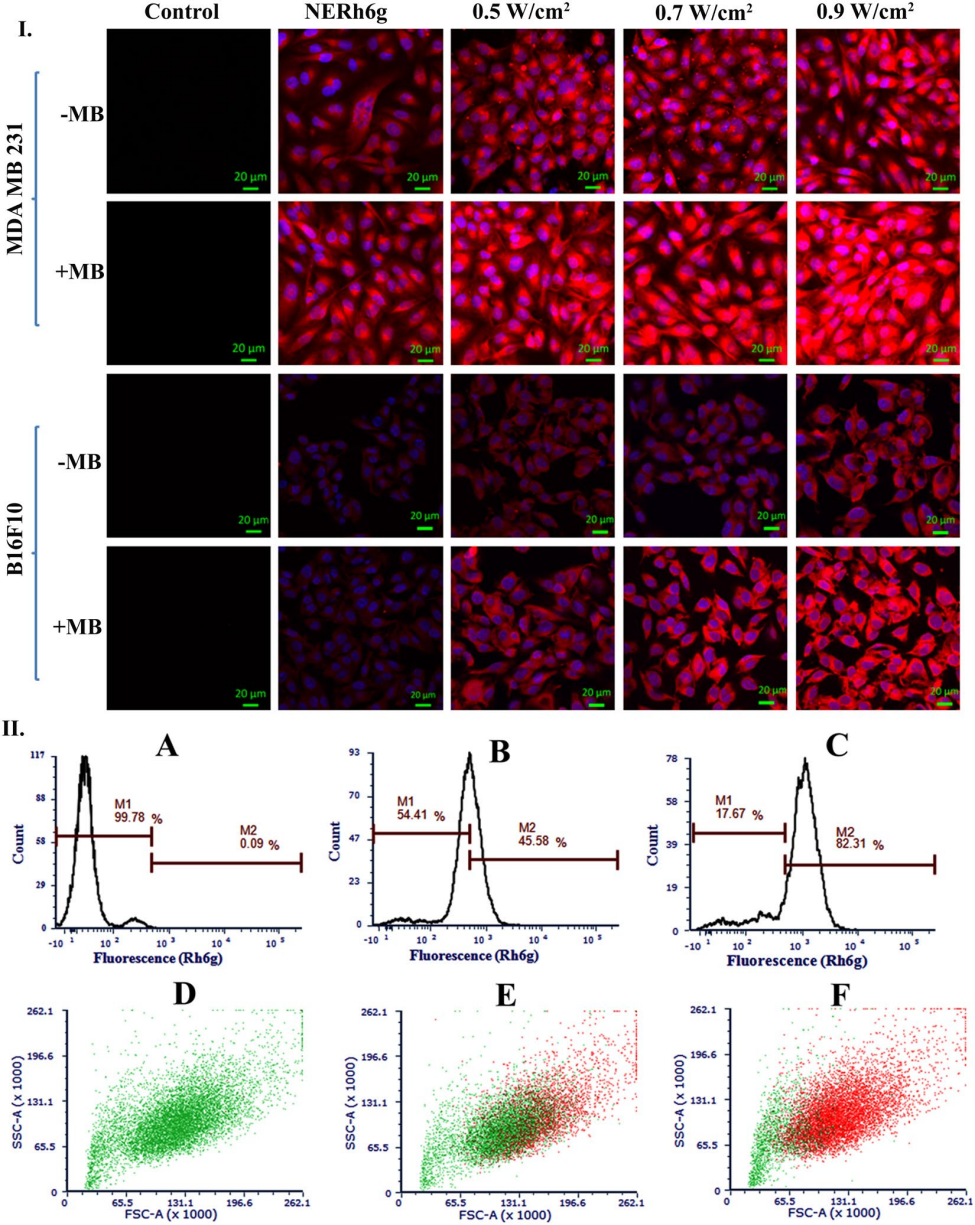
I. CLSM image of NERh6g internalized B16F10 melanoma and MDA MB 231 breast cancer cells. Cells were treated with different intensity of ultrasound in the presence and absence of MB: control, only NERh6g, NERh6g + 0.5 W/cm^2^, 0.7 W/cm^2^, and 0.9 W/cm^2^. II. Internalized NERh6g, by MDA MB 231 cells, was quantified by flow cytometry. Ultrasound treatment enhanced the amount of NERh6g inside cells. M1 indicates the NERh6g negative cells whereas M2 indicates NERh6g positive cells. In the lower panel, green dots indicate cells that are negative for NERh6g whereas the red dots are representing cells that are positive for the NERh6g. (A,D) Only cells, (B,E) cells were treated with NERh6g without ultrasound, (C,F) cells were treated with NERh6g and exposed to ultrasound at 0.9 W/cm^2^ intensity and 50% duty cycle for 30 sec.
